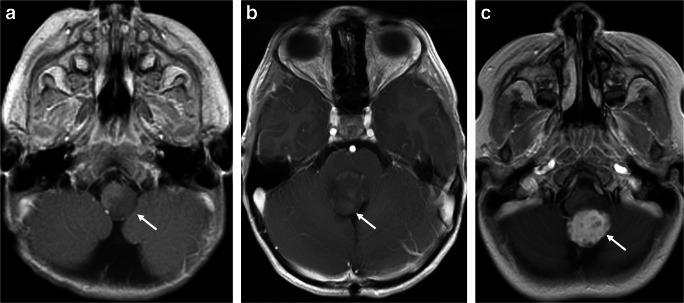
Enhancement patterns of dorsally exophytic brainstem glioma in three patients. a Axial post-contrast T1-weighted sequence through the medulla shows a nonenhancing (arrow) exophytic tumor arising from the left dorsal surface of the medulla in the first patient. b Axial post-contrast T1-weighted sequence through the pons in the second patient shows minimal patchy enhancement (arrow) of a dorsally exophytic tumor arising from the dorsal surface of the pons. c Axial post-contrast T1-weighted sequence through the medulla in the third patient shows an intensely enhancing (arrow) dorsally exophytic tumor arising from the dorsal surface of the medulla
